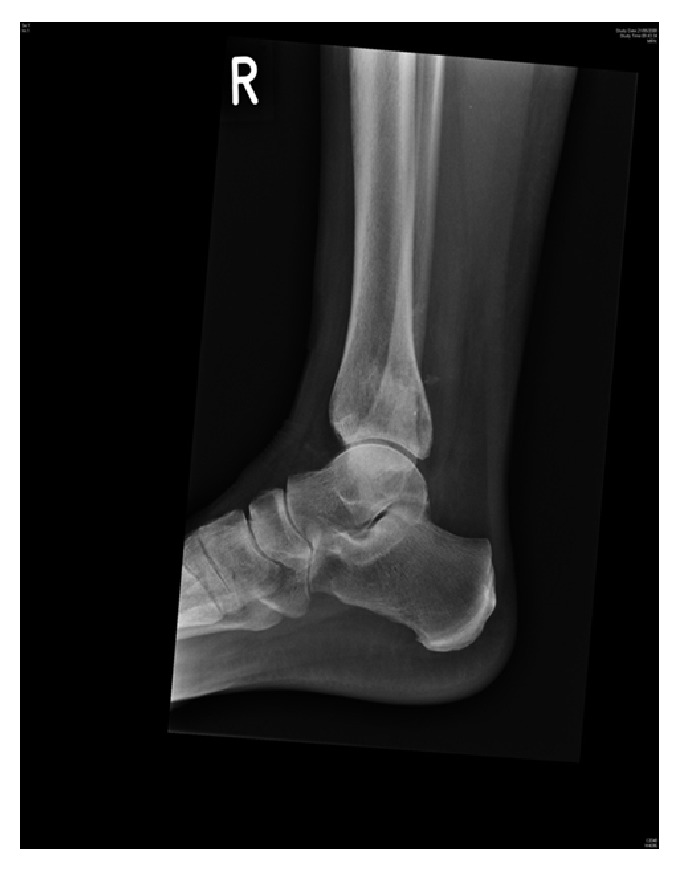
Lateral view X-ray at follow-up showing complete incorporation of graft and healing of lesion.
